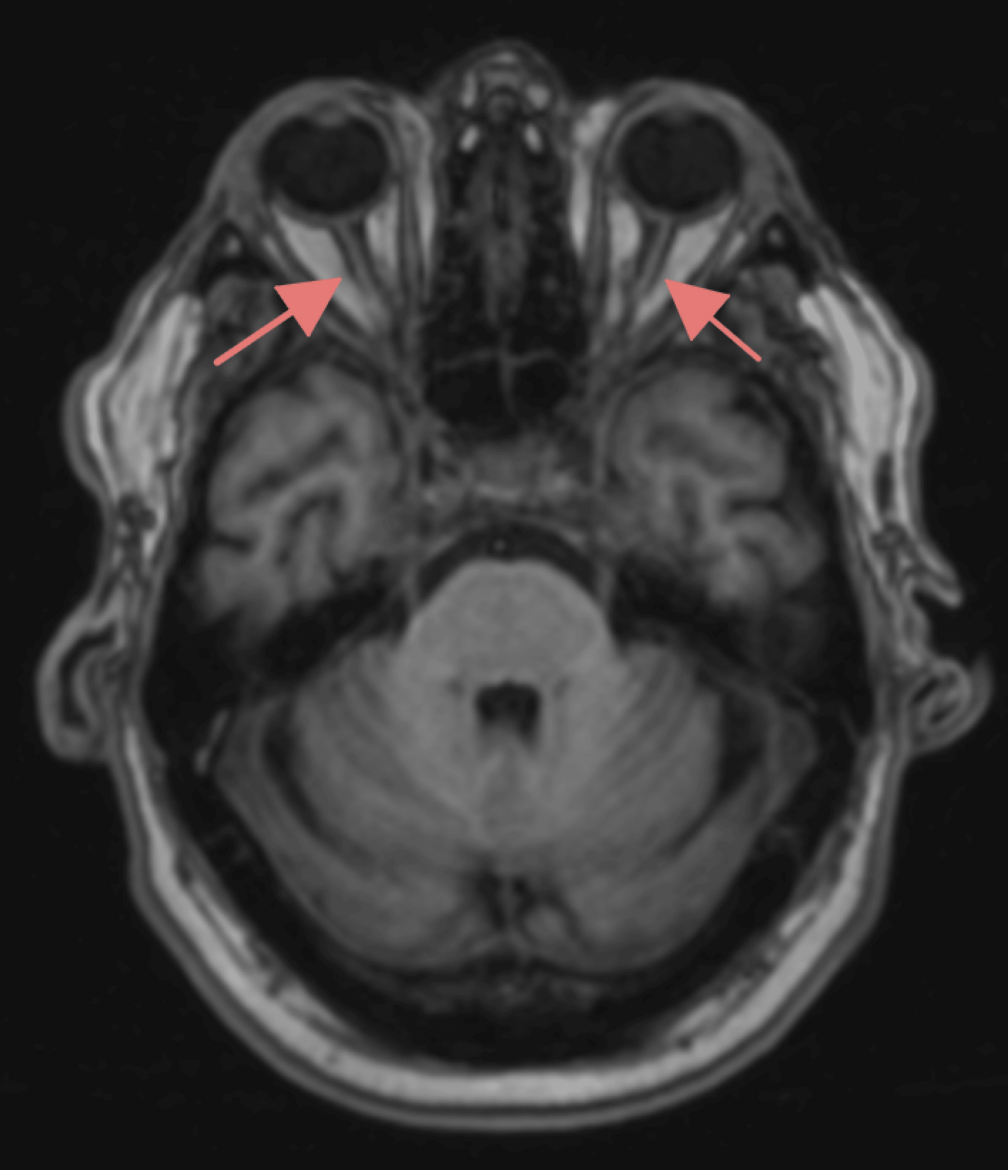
Axial T1-weighted MRI of the orbits Bilateral optic nerves appeared somewhat atrophied with diminutive caliber (red arrows). There is no enlargement of the extraocular muscles, arguing against thyroid ophthalmopathy. No acute ischemia or mass effect. MRI, magnetic resonance imaging
